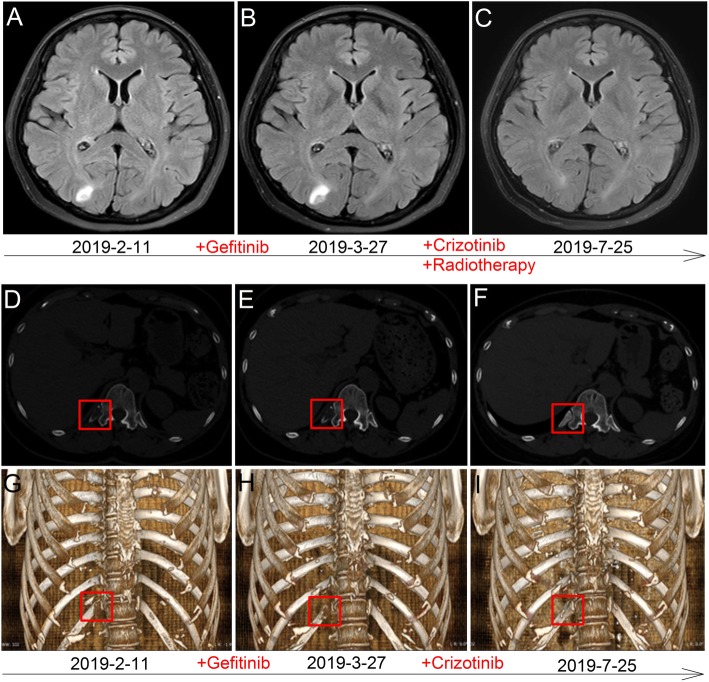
Management of brain and rib metastasis treatment. Head MRI (2019/2/11) showed a 13 mm cranial lesion (upper panel: T2-weighted MRI) (a) and bone destruction in the right posterior 12th rib was detected by an CT scan (d and g). After 1 months of EGFR tyrosine kinase inhibitor treatment, a slight enlargement (to 16 cm) of the brain metastasis lesion (b) and bone destruction in 12th rib worsened(e and h). The patient was treated with crizotinib, brain metastatic lesion received a local tomotherpy contemporary but the rib lesion without radiotherapy. Three months after the therapy, imaging examinations revealed obvious decrease in the size of the brain metastases (c). The rib lesion almost disappeared and showed bony restoration (f and 2i)
